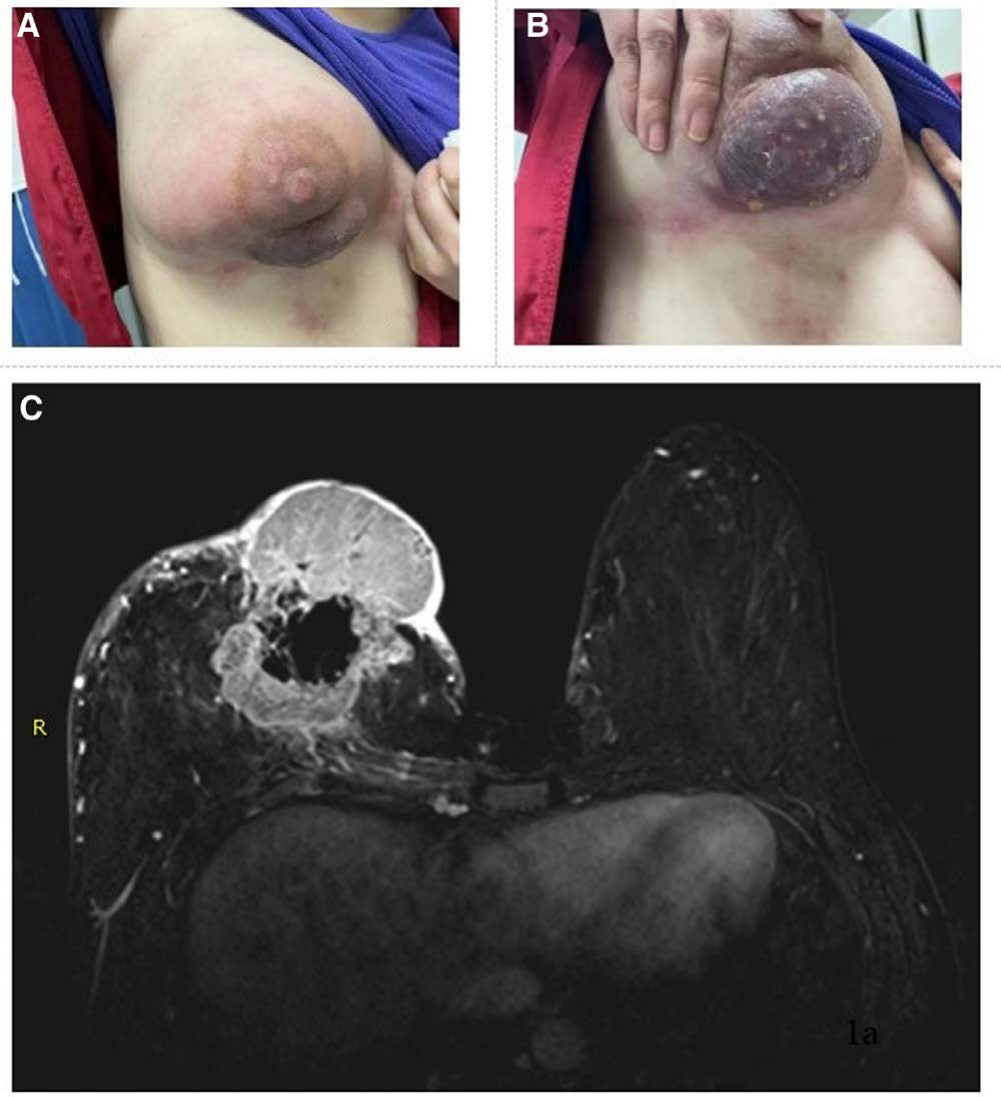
(A and B) Appearance of the breast mass before the treatment. (C) Enhanced MRI of the breast before the treatment. MRI = magnetic resonance imaging.
